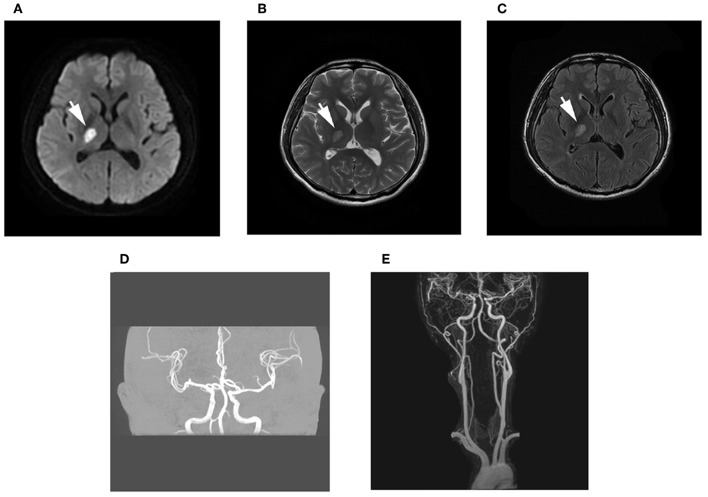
Brain magnetic resonance imaging (MRI). (A–C) Diffusion-weighted imaging (DWI) and T2 fluid-attenuated inversion recovery (FLAIR) imaging showed high signal, while long signal was shown in T1-weighted imaging and T2-weighted imaging of the posterior limb of the internal capsule. (D,E) Magnetic resonance angiography examination of the intracranial and cervical vascular was normal.
